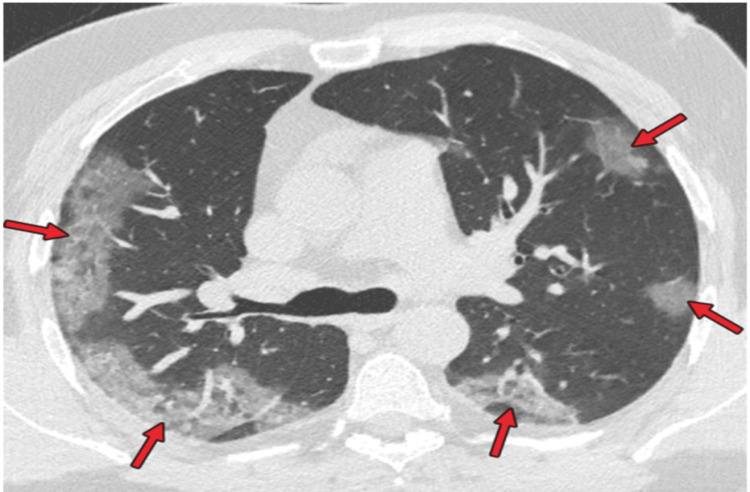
Axial non-enhanced chest CT image The image shows the bilateral and peripheral distribution of ground-glass opacities (GGOs) (arrows) in COVID-19 pneumonia. Image source: Reference no. [15]
